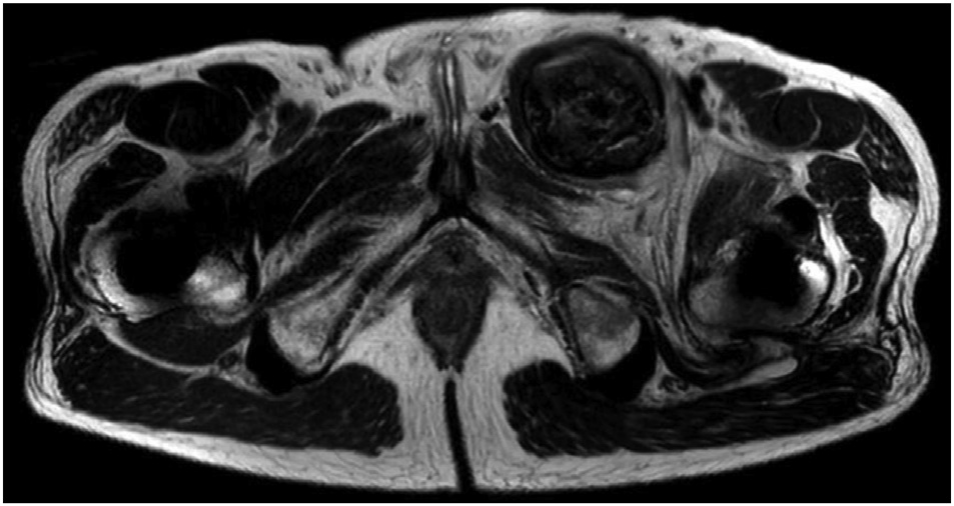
Axial T2 MARS-MRI pelvis with contrast demonstrates pseudotumor formation within the left anterior pelvis and bilateral peritrochanteric fluid signal. MARS = magnetic artifact reduction sequence.
